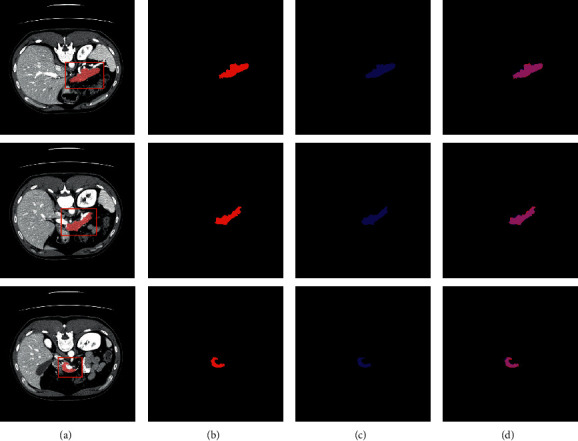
Comparisons of 2D pancreas segmentations from the proposed DUNet with the manual segmentations. The first, second, and third columns denote the CT slices with their segmentations and bounding boxes of pancreas (red), the manual segmentations, and the network predictions, respectively. The last column denotes the overlapped maps between the network predictions and manual segmentations, with overlapped regions marked by magenta. (a) Original. (b) Groundtruth. (c) Prediction. (d) Overlapped.
